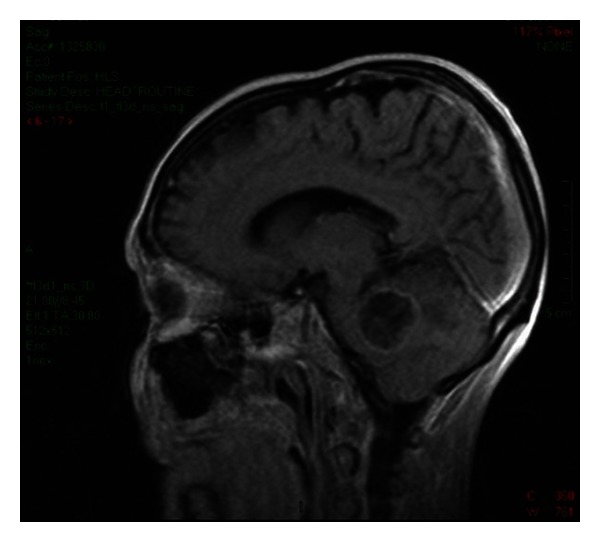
Cystic metastasis. Infratentorial, rim-enhanced hypointense lesion revealed by post-contrast T1-weighted MRI image. Department of Neurology, Colentina Clinical Hospital brain imaging archive.
